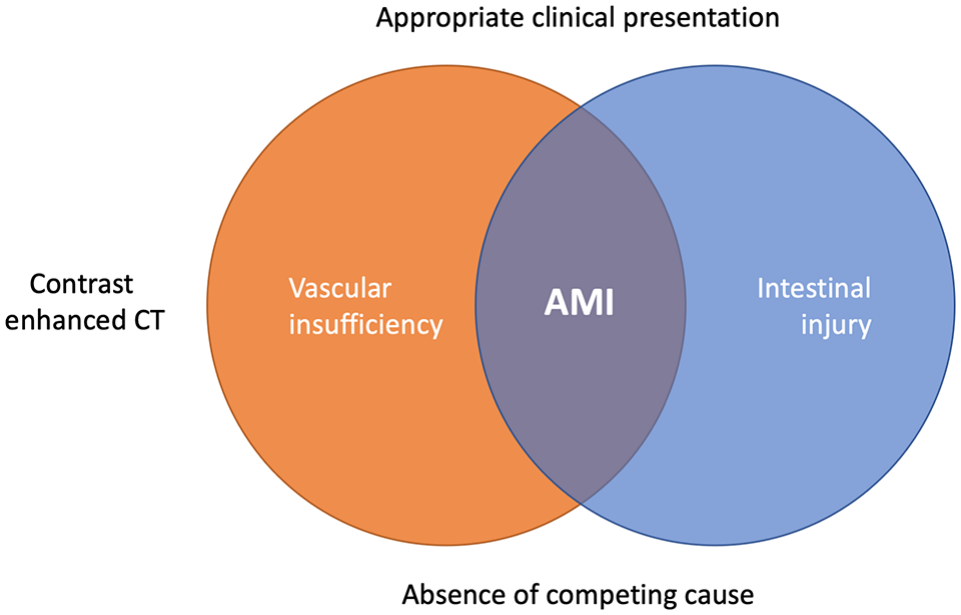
The diagnosis of acute mesenteric ischemia (AMI) is based on findings of vascular insufficiency and signs of intestinal injury in contrast-enhanced computed tomography (CT) accompanied with appropriate clinical presentation.
